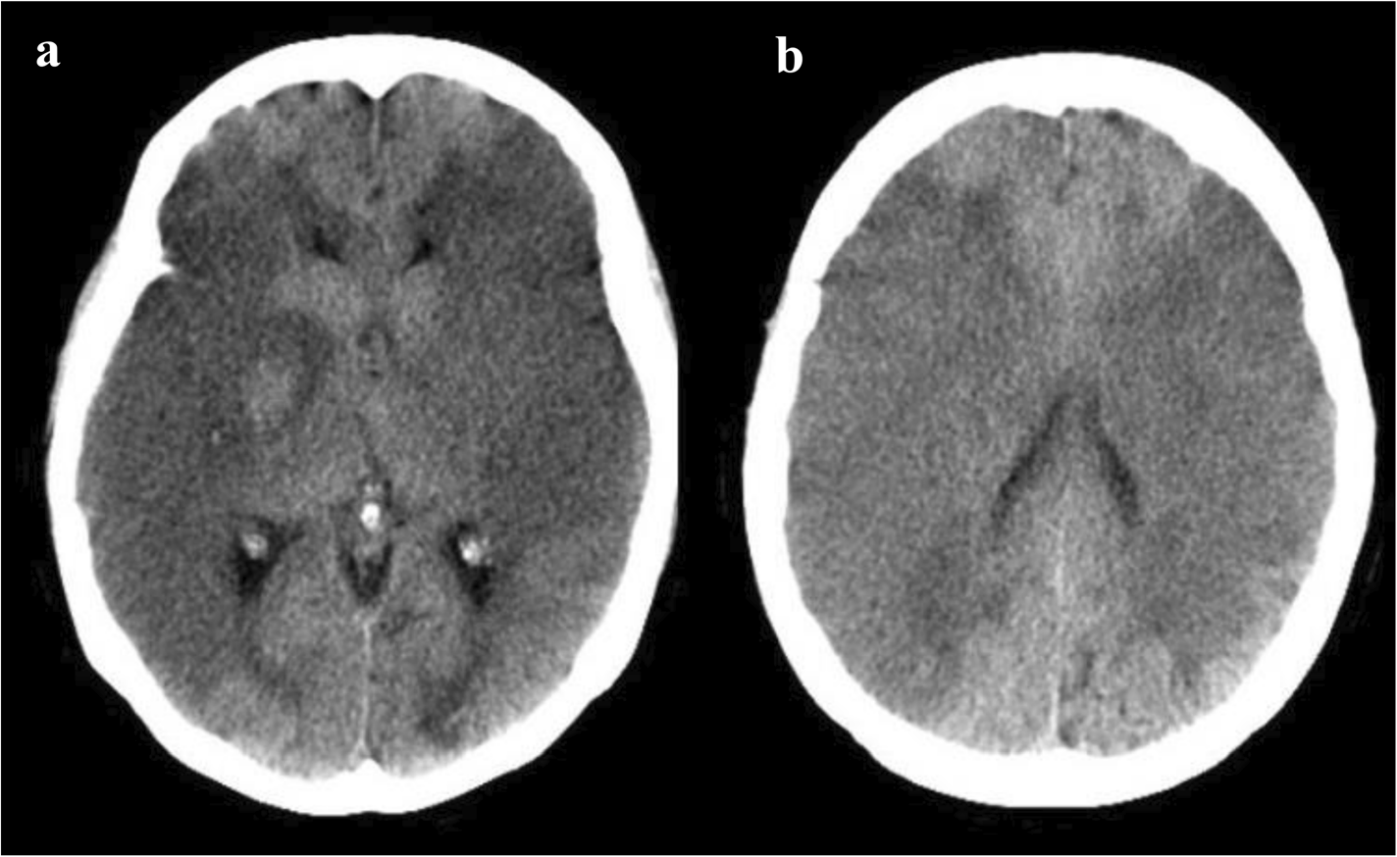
Computed tomography of the brain. a Decrease in attenuation and loss of gray-white differentiation of bilateral cerebral hemispheres supplied by the middle cerebral artery territories, with a narrowing of the bilateral lateral ventricle due to compression by swelling brain parenchyma. b The infarction in the right and left middle cerebral artery distribution, with sparing of the bilateral frontal and occipital lobes
